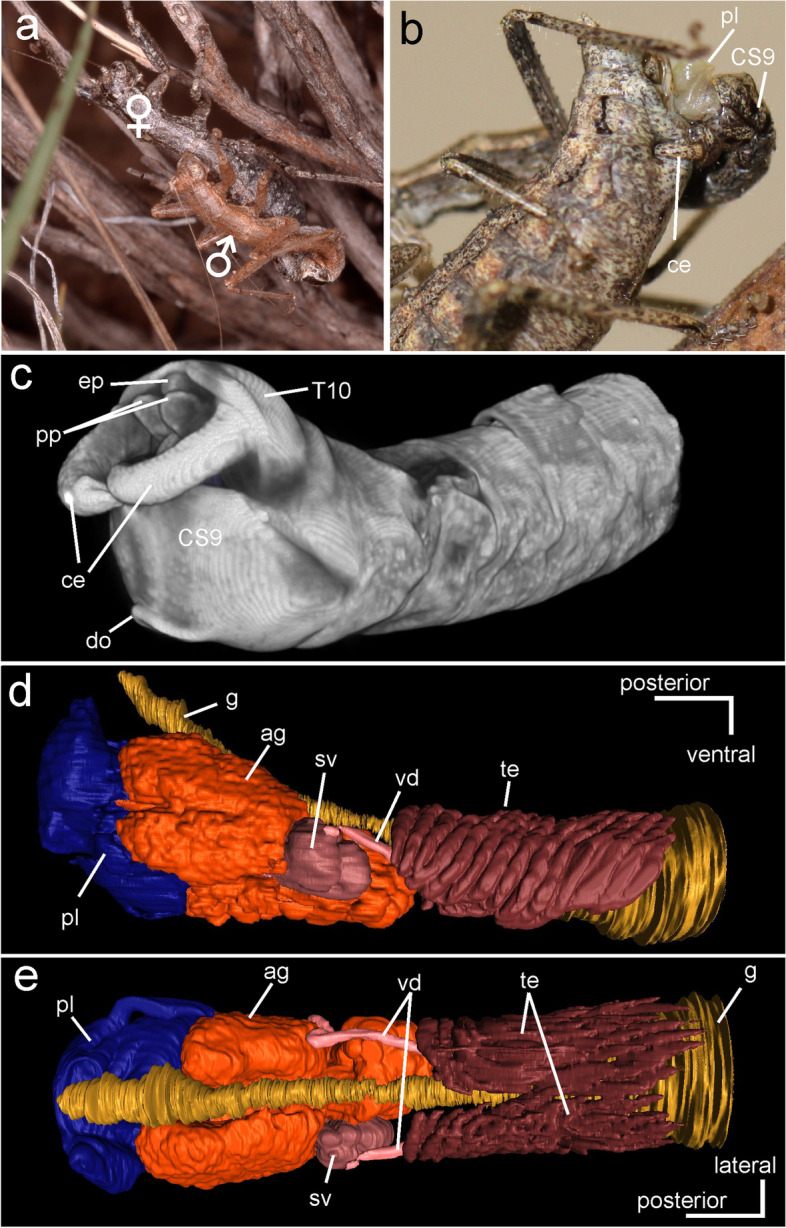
a Mating couple exhibiting the false-male-above-mating position in *Karoophasma biedouwense* (Mantophasmatodea). The male sits above the female and bends its abdomen in an S-shape around her right side, bending its abdomen to the left to insert external genitalia into the female genital opening (photo by Mike D. Picker). b Close-up of the copulation position during the insertion process. Note the cerci which are used as claspers to hold on to the female abdomen, while the membranous phallic organ is inserted. c μ-CT-based volume rendered reconstructed postabdomen of a male *K. biedouwense*. d-e μ-CT-based 3D reconstructions of internal reproductive organs and gut from lateral (d) and dorsal view (e). ag – accessory gland, CS9 – abdominal coxosternum IX (subgenital plate), ce – cerci, do – drumming organ, ep – epiproct, g – gut, pl – phallic lobes, pp – paraproct, sv – seminal vesicle, T10 – abdominal tergum X, te – testis, vd – vas deferens
